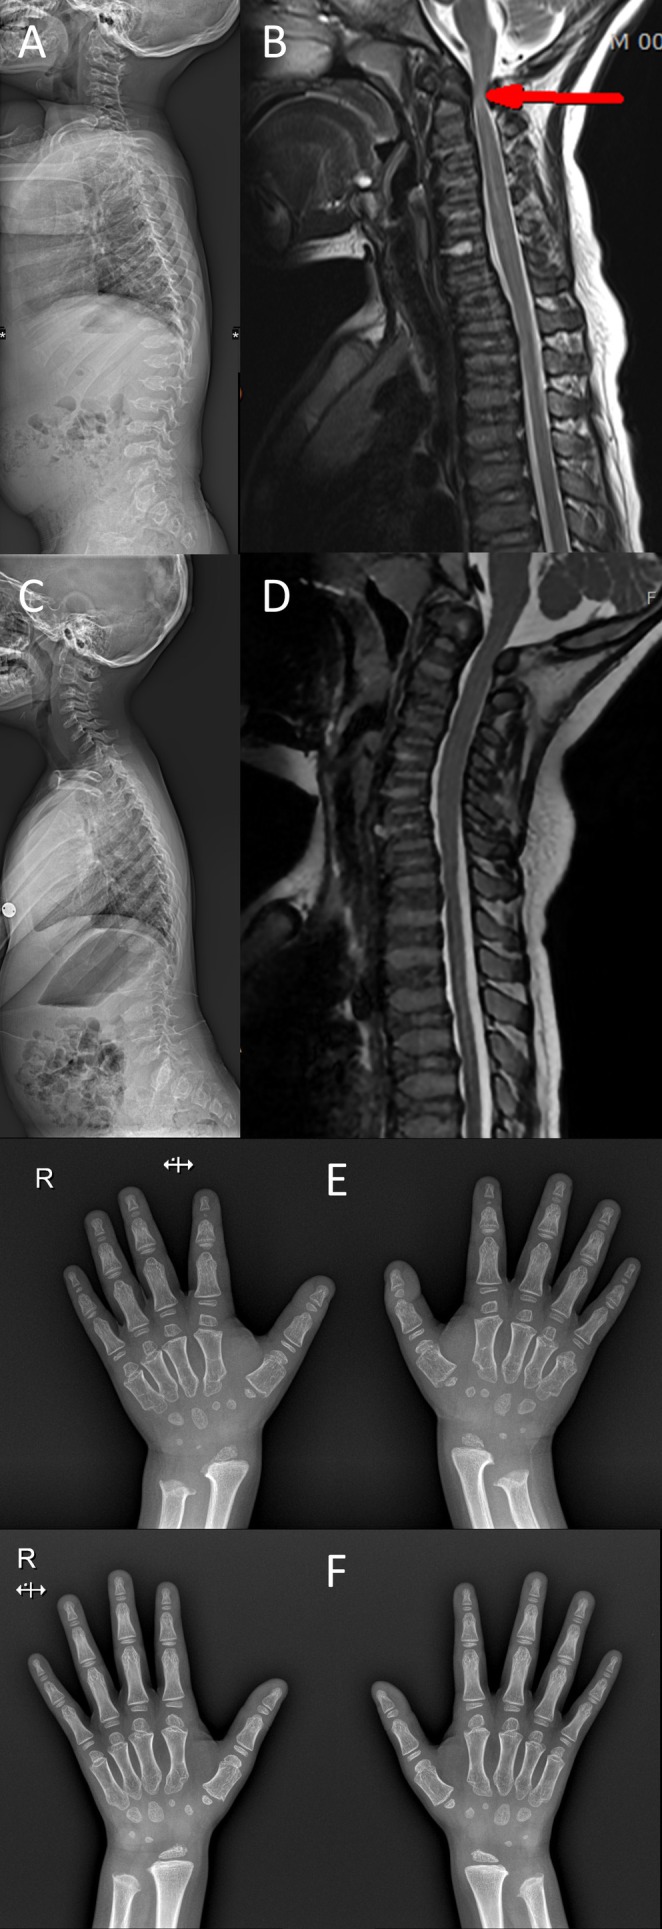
Radiographs and spinal magnetic resonance imaging (MRI) of siblings with mucopolysaccharidosis IVA. (A) Spinal column radiograph (Sibling 1, 5.4 years). (B) Spinal MRI (Sibling 1, 5.2 years) showing spinal cord compression at the cervicomedullary junction (C1 level). (C) Spinal column radiograph (Sibling 2, 5.2 years). (D) Spinal MRI (Sibling 2, 4.7 years) showing no spinal cord compression. (E) Hand radiograph (Sibling 1, 5.0 years). (F) Hand radiograph (Sibling 2, 4.2 years).
